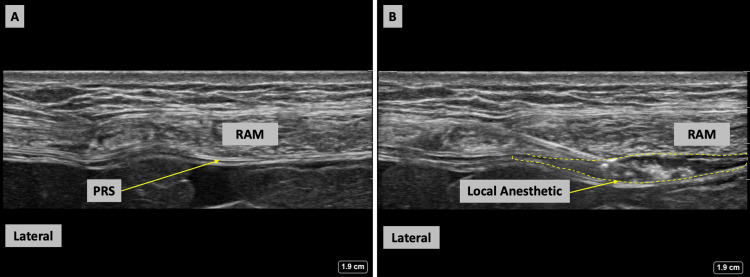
Ultrasound images of the rectus sheath injection technique (A) Before injection of local anesthetic, the RAM and the PRS are labeled. (B) The nerve block needle is advanced in-plane in a lateral to a medial trajectory and can be seen piercing the posterior border of RAM. Local anesthetic is deposited between the RAM and PRS and can be seen expanding this fascial plane. RAM = rectus abdominis muscle, PRS = posterior rectus sheath
